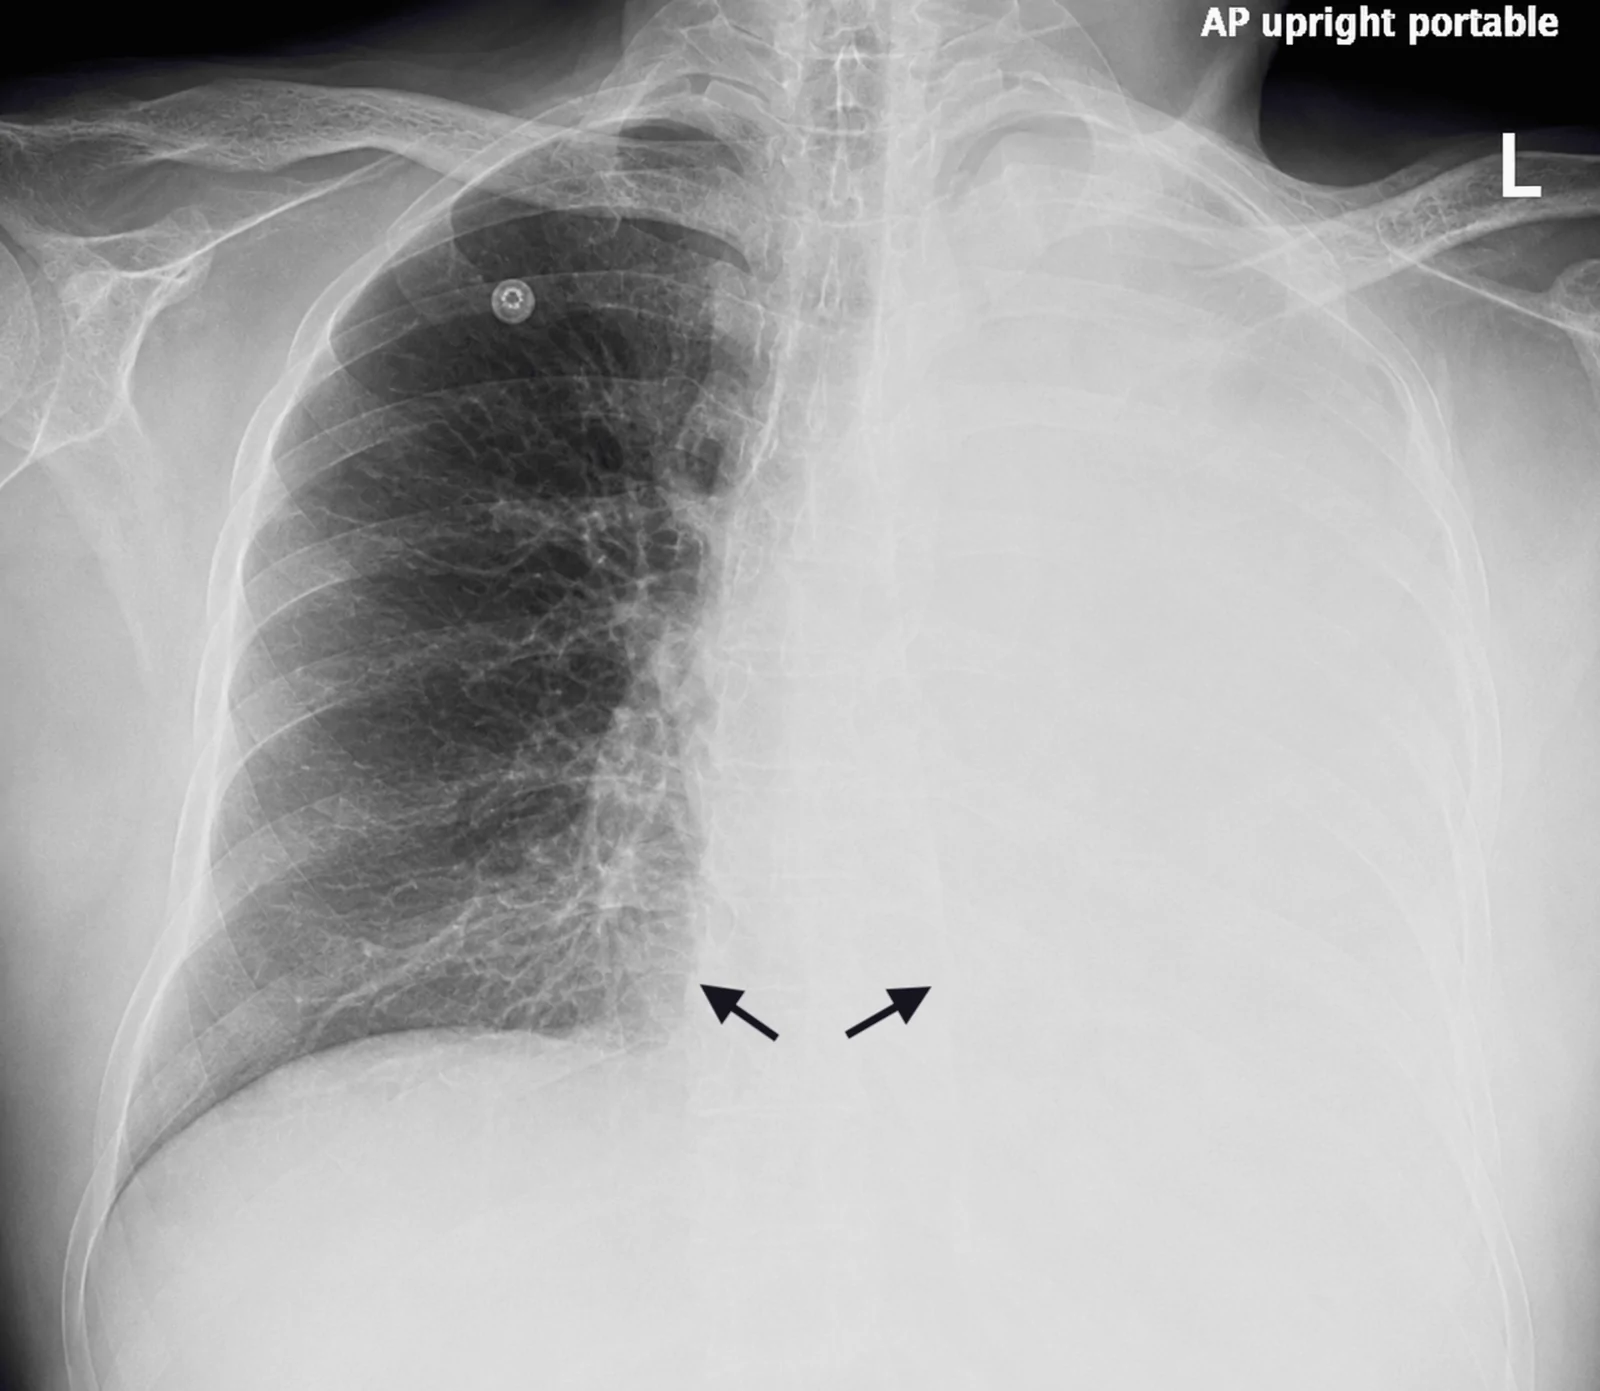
Chest radiograph obtained on hospital Day 2 Demonstrating persistent large left and moderate right pleural effusions with near-complete collapse/consolidation of the left lung. Persistent pleural abnormalities in the setting of fever, leukocytosis, and progressive respiratory distress prompted diagnostic thoracentesis.
